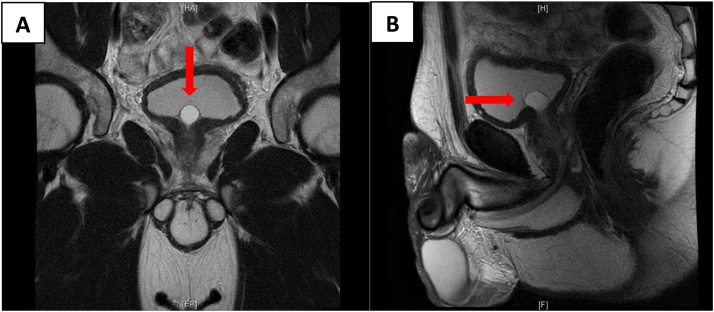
Coronal (A) and sagittal (B) pelvic MR images of the male patient showing a well-defined midline cyst arising from the prostatic base and herniating into the urinary bladder with no clear communication with the urethra. The cyst measured 1.6*1.3 cm and exhibited bright T2 signal intensity and hypo intense T1 signal intensity with peripheral wall enhancement characteristic of a cyst (red arrow).
